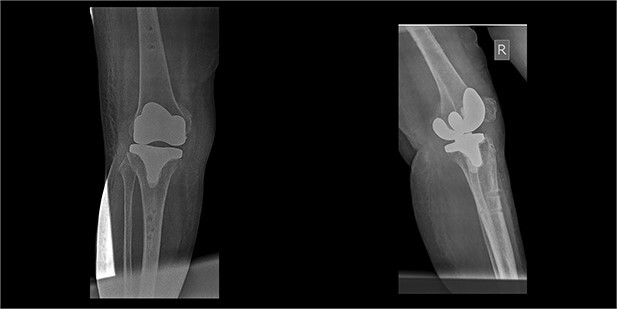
X-rays 3-weeks post removal of external fixator.
